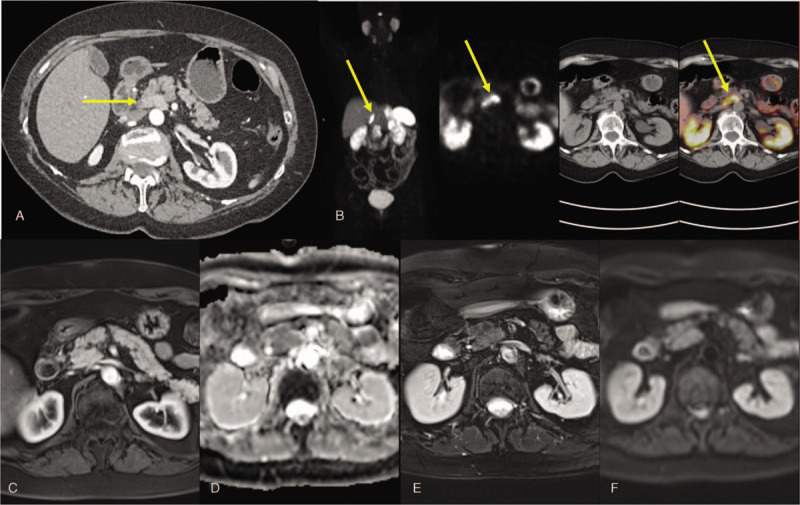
(A) Computed tomography (CT) scan showing mild enhancement in the pancreatic uncinate process. (B) ^68^Ga DOTATATE positron emission tomography (PET)/CT imaging demonstrating uptake in the pancreatic uncinate process. (C–F) MRI with no corresponding anatomic abnormality on (C) T1 post-contrast, (D) apparent diffusion coefficient (ADC), (E) T2-weighted fat suppressed, and (F) diffusion-weighted imaging (DWI).
